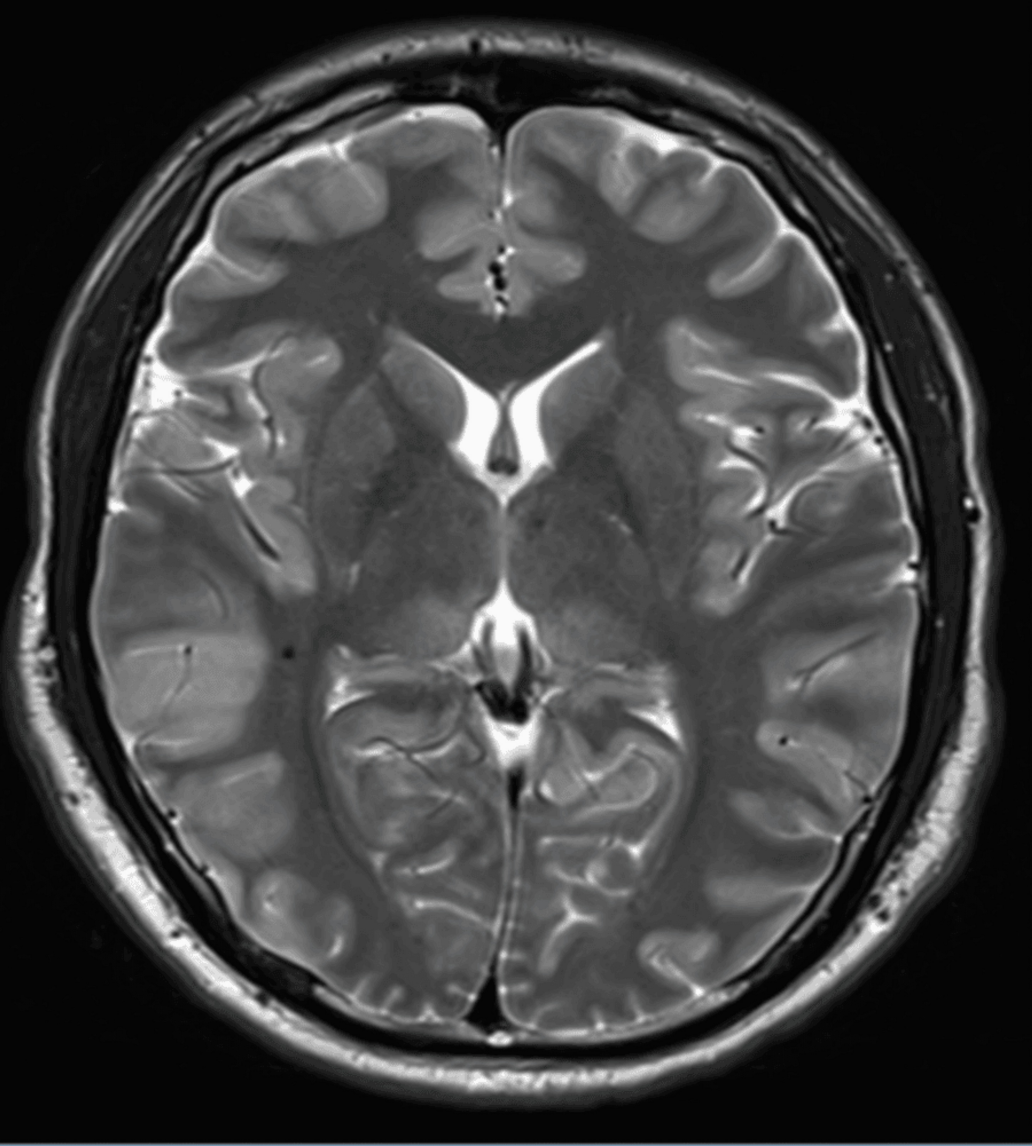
MRI brain; diffuse cytotoxic edema secondary to diffuse anoxic brain injury
